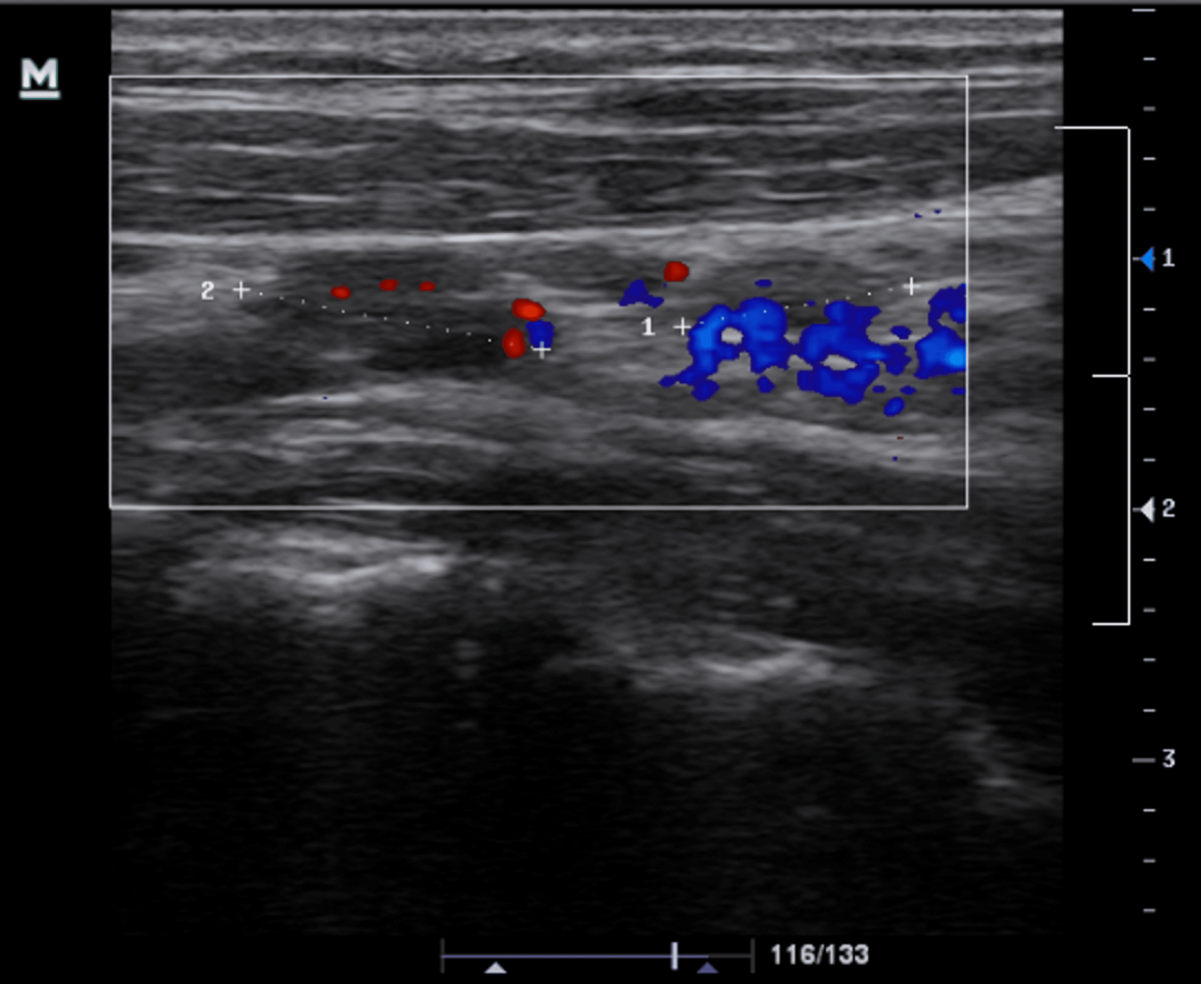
Bilateral lymphadenopathy was demonstrated on ultrasound on the initial visit.
